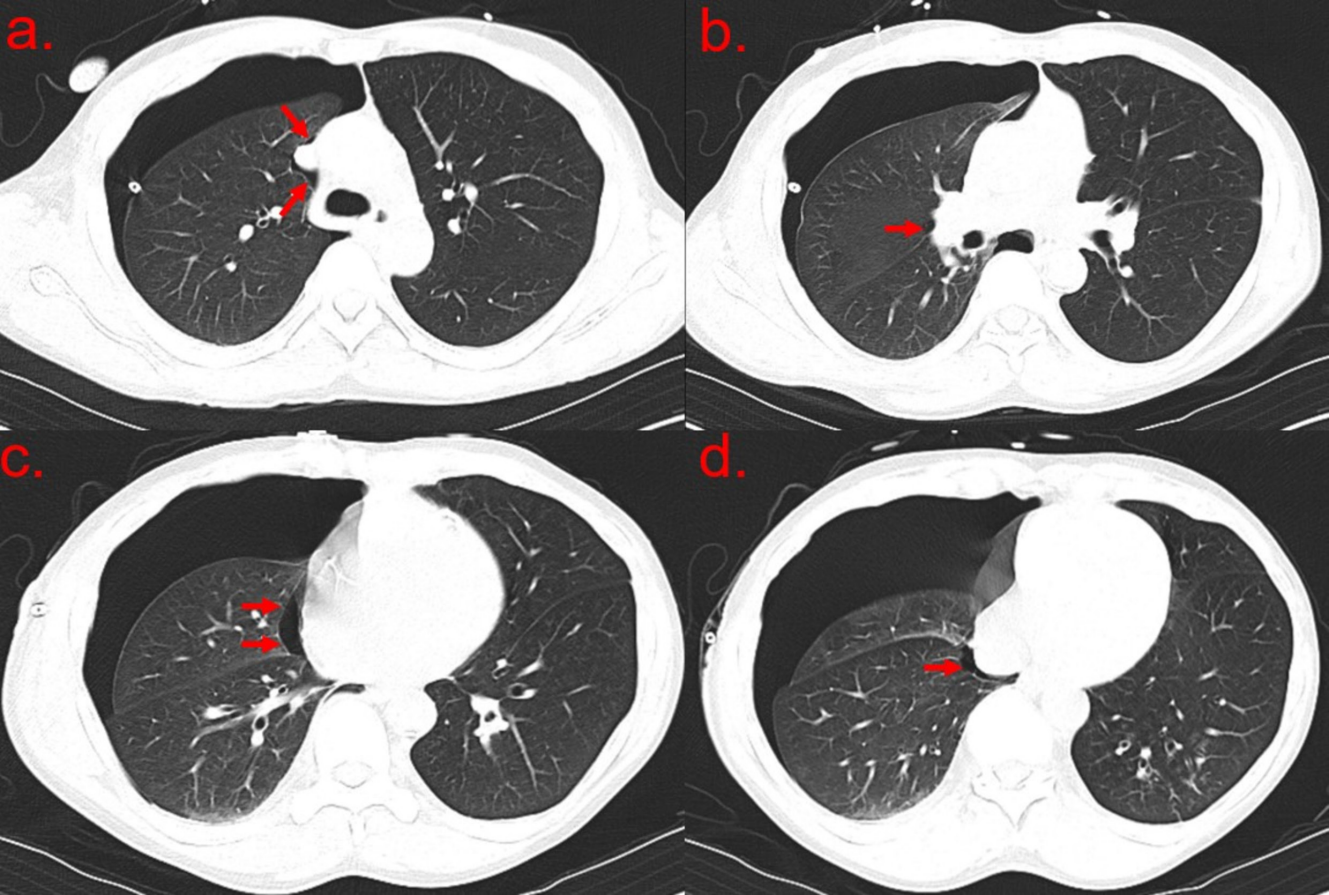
Pre‐operative CT revealing blebs and bullae (red arrows).
